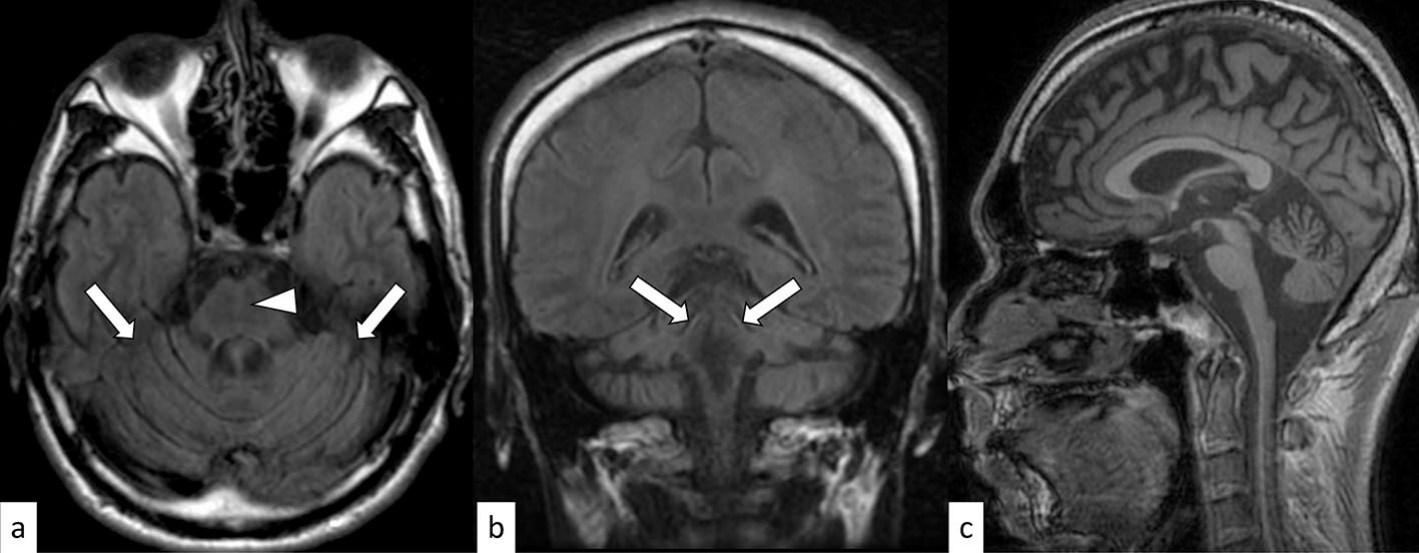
A 48-year-old man with SCA1. FLAIR axial image a and FLAIR coronal image b show cerebellar atrophy including superior cerebellar peduncles (arrows) and a vertical hyperintense line reflecting pontine atrophy (an incomplete hot cross bun sign) (a, arrowhead). T1-weighted sagittal image shows an atrophy of the brainstem and cerebellum (c)
